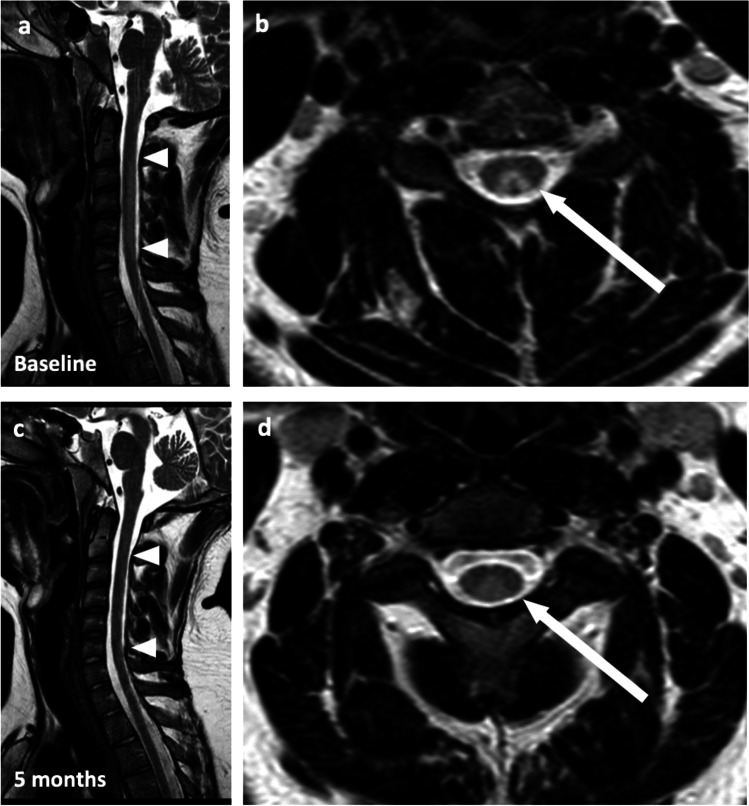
a–d T2-weighted sagittal mDixon sequences and axial TSE sequences of the cervical myelon. The baseline shows sagittal dorsal long-range signal enhancements (cervical vertebra C2-6; triangle) of the myelon (a). In the axial (b) sequences, they are prominent in the posterior strands (“V-shaped,” arrow). After 5 months of vitamin B12 substitution, lesions (arrow) have regressed (d). At cervical vertebra C2-6, a residual is found with remaining flat signal elevations (triangle) of the dorsal spinal cord section (c)
